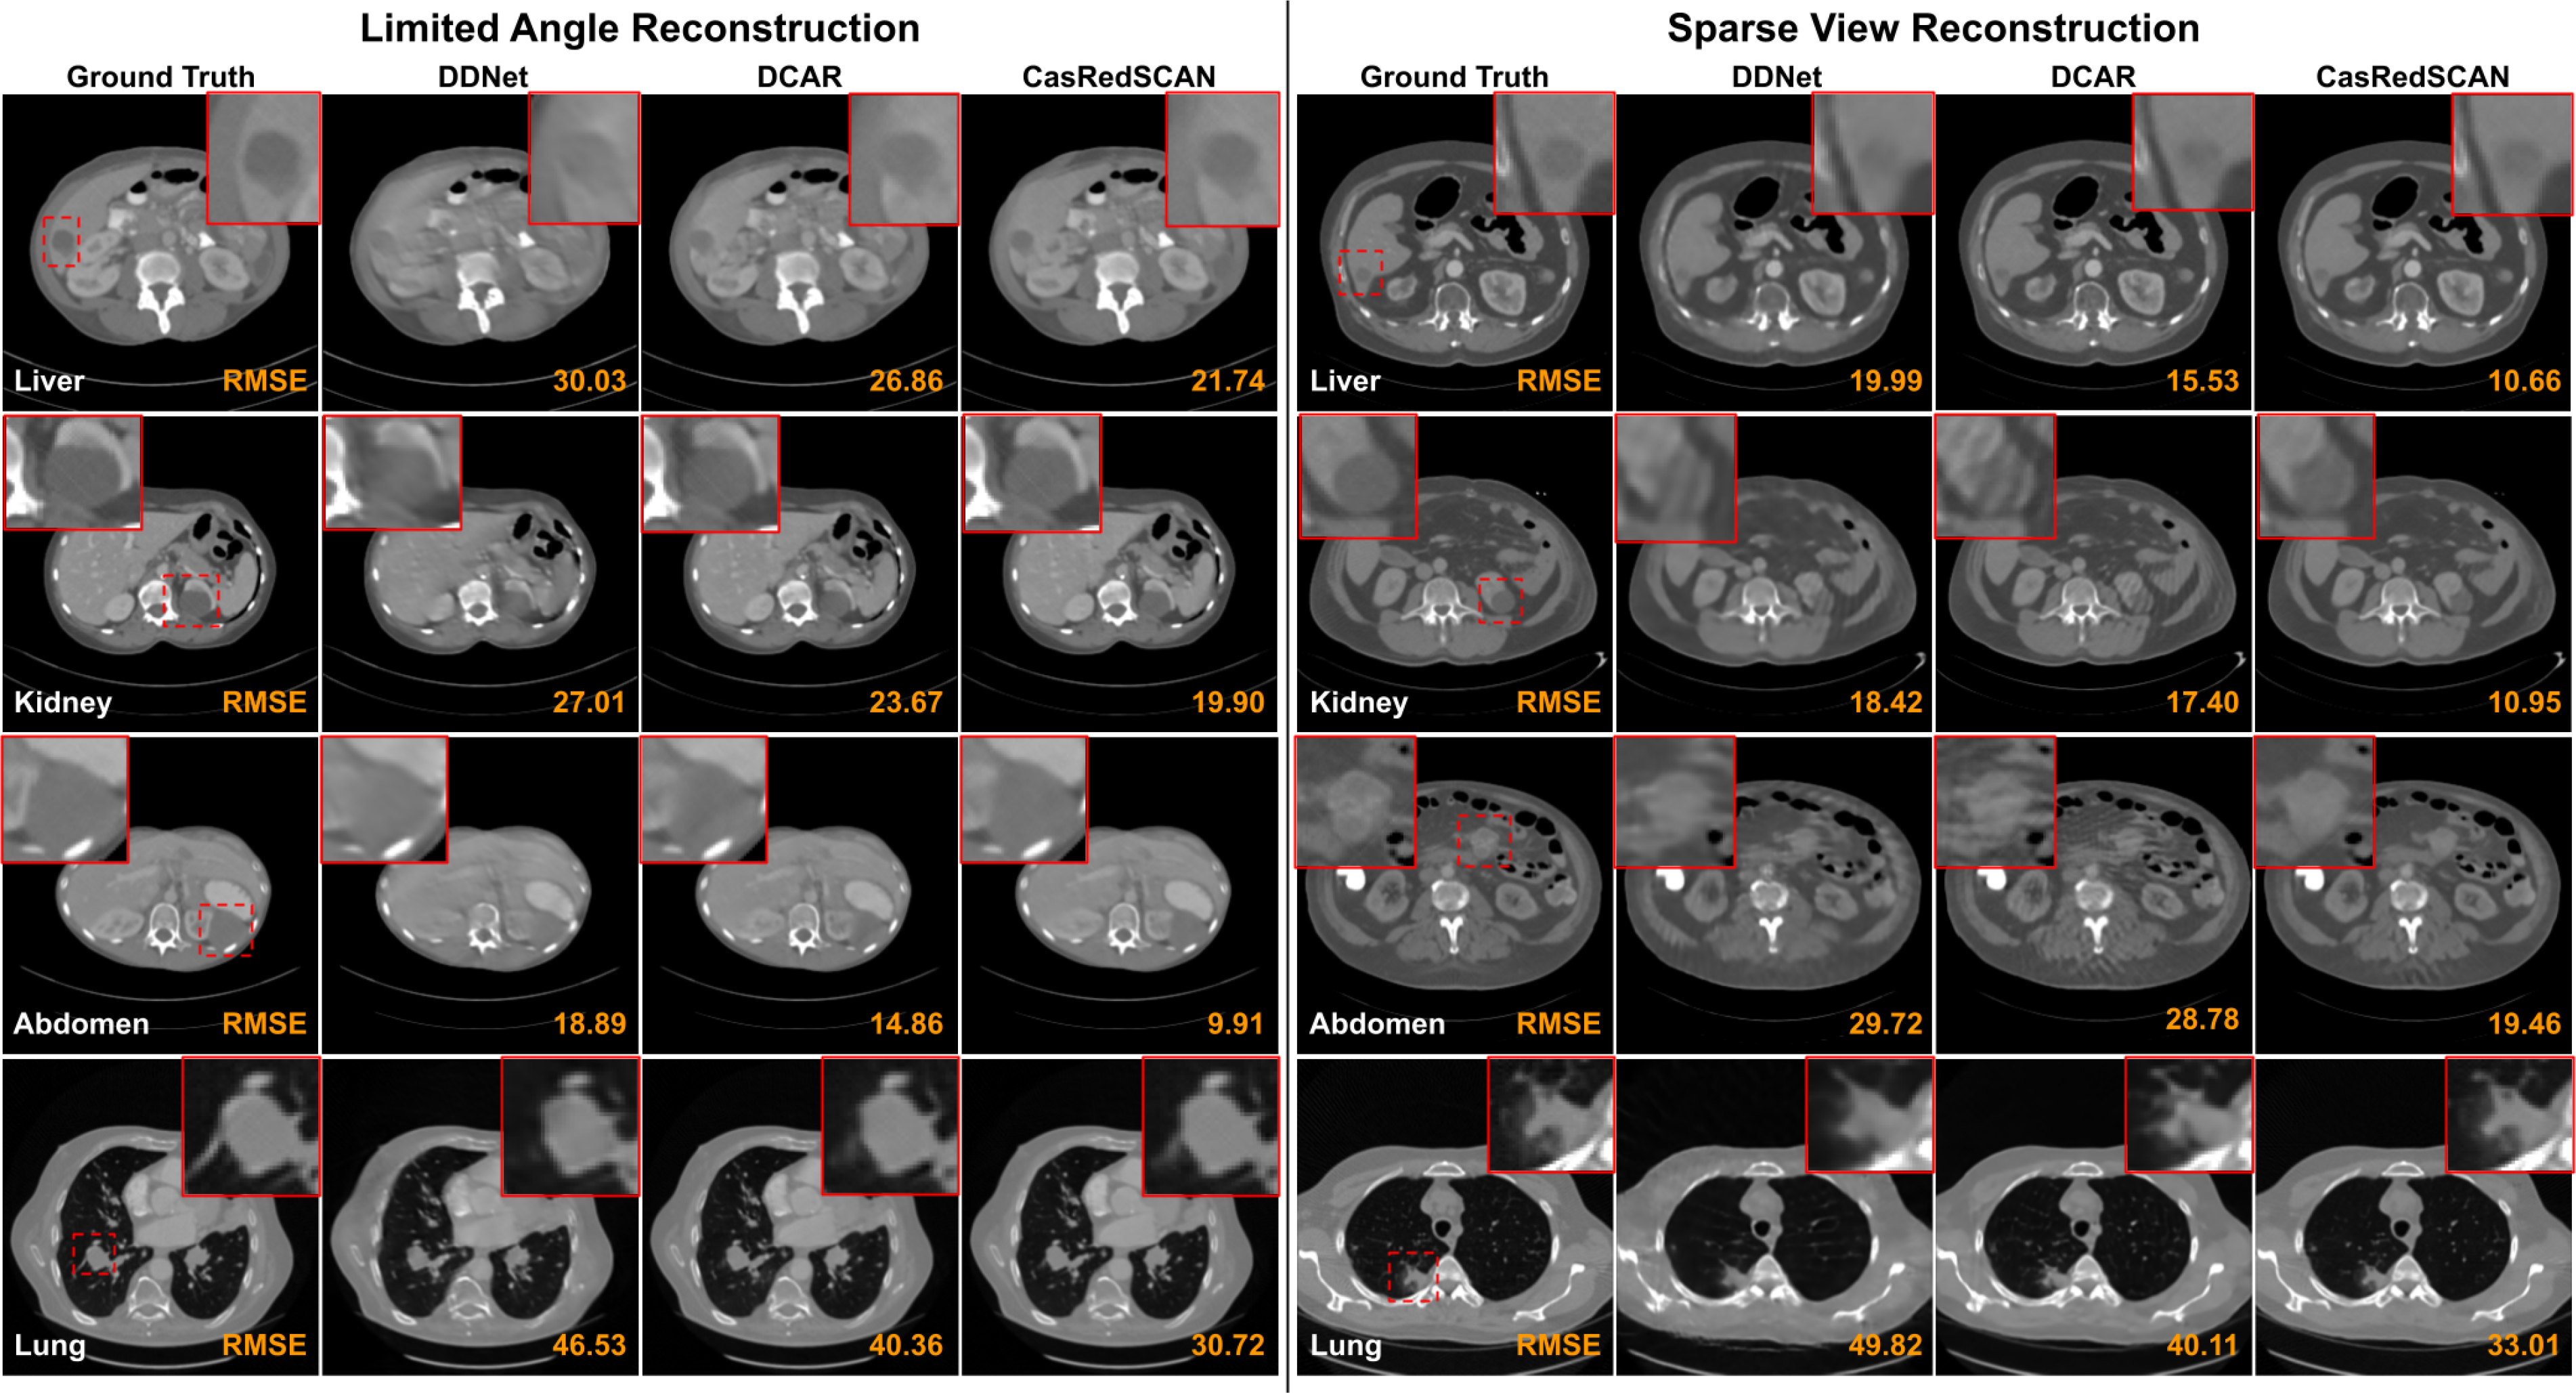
Comparison of limited angle reconstructions (120° limited angle) and sparse view reconstructions (1/4 downsampling) in CT scans with lesions. The lesion region zoom-in views are shown on the top. The display window of liver, kidney, and abdomen CT is [−300 500] HU. The display window of lung CT is [−1000 1000] HU.
